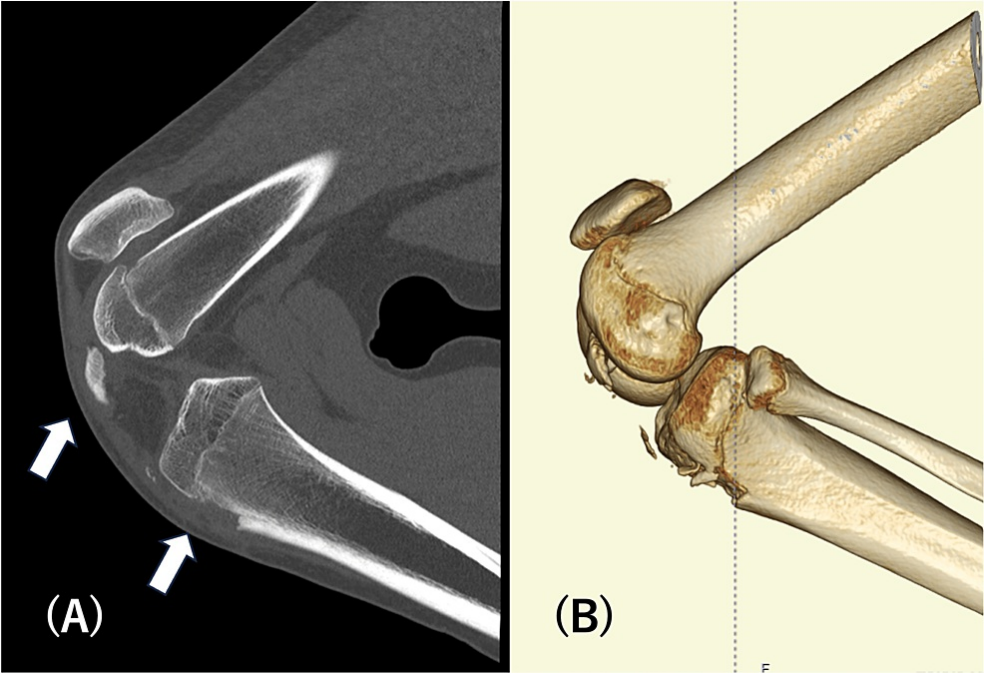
Preoperative computed tomography images show no sign of intra-articular involvement.
